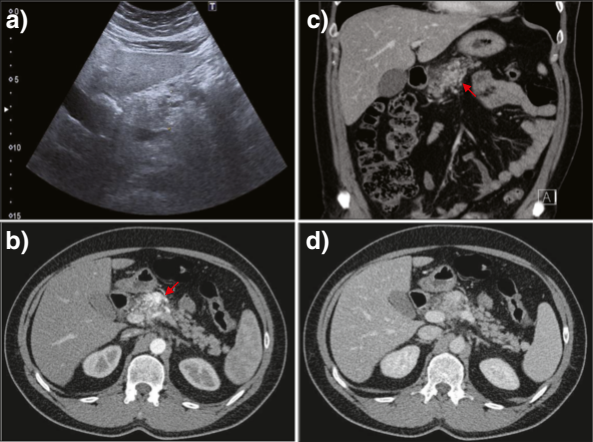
US imaging showed a structurally heterogeneous and thickened neck of the pancreas, hardly differentiated from the surrounding fat and without any dilatation of the main pancreatic duct (a). Contrast-enhanced CT examination through the pancreas demonstrated a mosaic hypervascular area at the same site as the initial US (b–d, *red arrows*)
